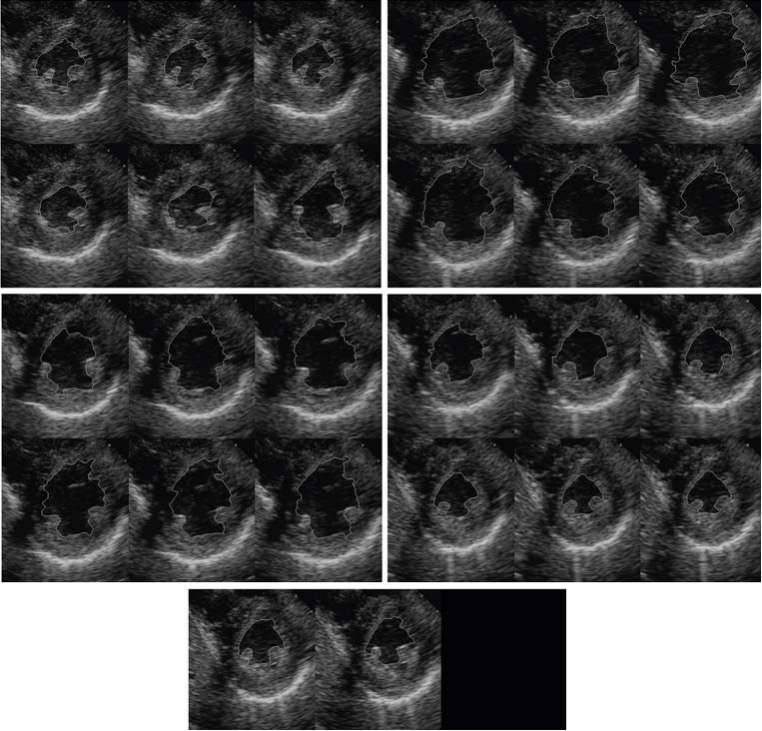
Parasternal short axis sequence segmentation using the new, hybrid method.
